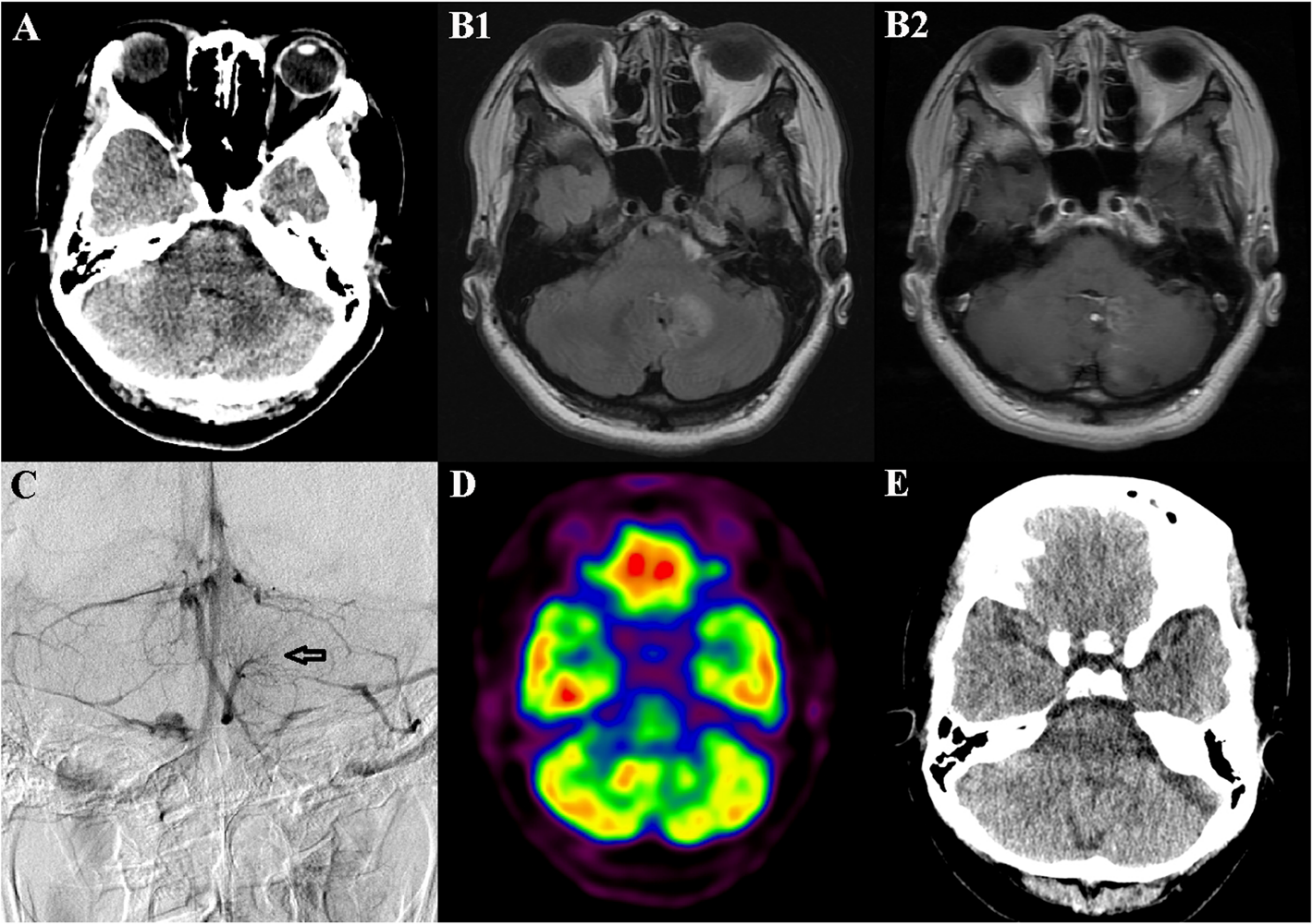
Brain imaging results. a: A non-contrast computed tomography (CT) image shows a hypodense lesion in the left cerebellar deep nuclei. b: A fluid-attenuated inversion recovery image shows vasogenic edema within the deep white matter of the left cerebellum, especially affecting the left dentate nucleus (b1). These signal abnormalities are observed in multiple enhancing medullary veins with caput medusa appearance in a gadolinium-enhanced T1-weighted axial image (b2). c: Venous phase of the left vertebral artery digital subtraction angiography in anteroposterior view demonstrates umbrella-shaped collections of dilated medullary veins in the left cerebellum (arrow). d: Asymmetrically reduced glucose metabolism in the affected left cerebellum is revealed in ^18^F-fluorodeoxyglucose positron emission tomography. e: A follow-up brain CT scan 1 month later shows resolution of the cerebellar edema
